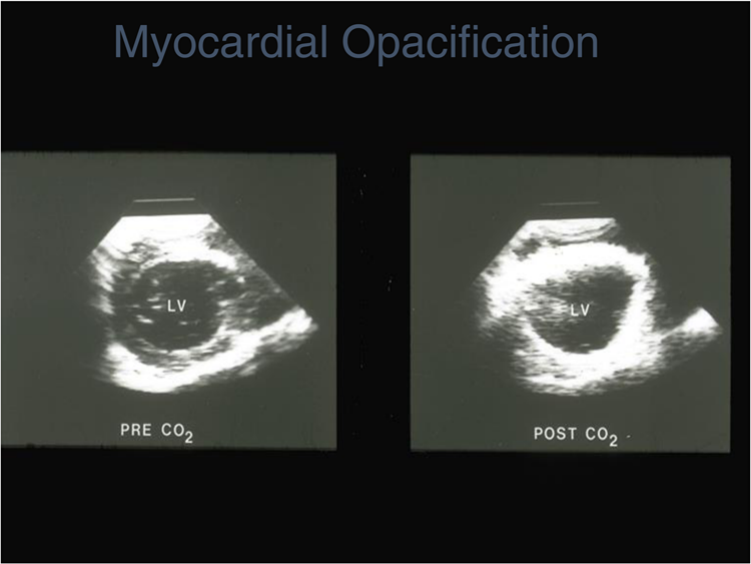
Images from a preclinical study in the parasternal short axis view before (left) and following (right) the intracoronary injection CO_2_
